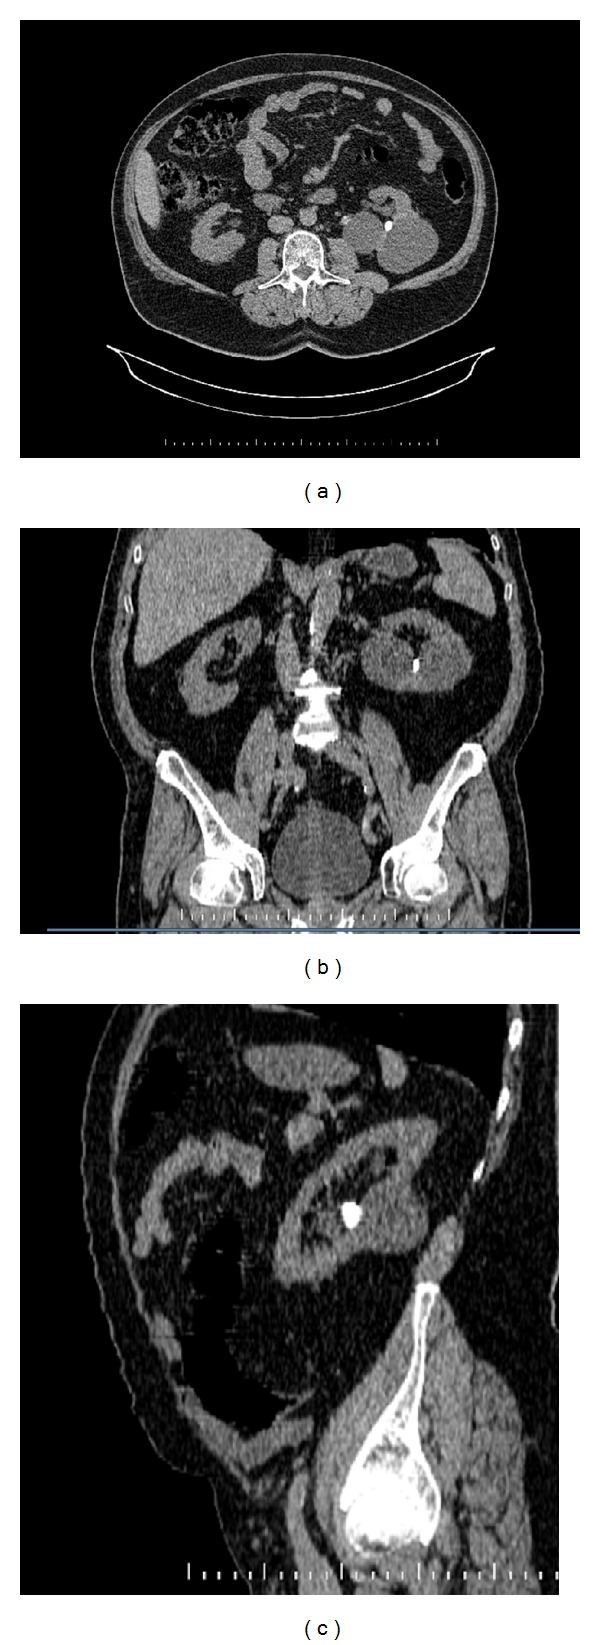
CT images of a patient with cysts and stone ((a) transvers, (b) coronal, and (c) sagittal).
